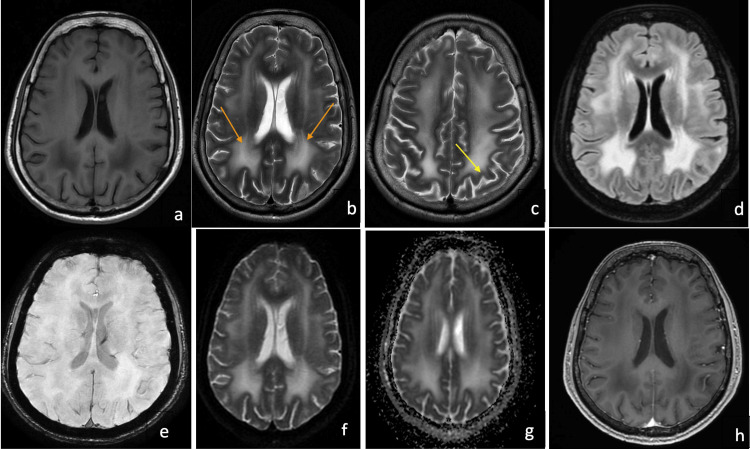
Magnetic resonance imaging (MRI) of the brain in the index case 1a. T1 weighted axial MRI of the brain showing bilateral symmetrical hypointensity in the periventricular white matter, 1b. T2 weighted axial MRI of the brain showing bilateral symmetrical hyperintensities in the periventricular white matter (orange arrows), 1c. T2 axial MRI brain highlighting the involvement of the subcortical U-fibers (yellow arrow), 1d. FLAIR hyperintensities noted in the bilateral periventricular white matter, 1e. Susceptibility-weighted imaging (SWI) showed no evidence of microbleeds, 1f. and 1g. Diffusion-weighted imaging (DWI) and attenuation diffusion coefficient (ADC) images demonstrate hyperintense signals in the periventricular white matter suggesting a T2 shine-through effect (probably due to vasogenic edema resulting from hyperpermeability of blood capillaries secondary to LAMA2 protein defect), 1h. T1 contrast images demonstrate the lack of contrast enhancement in the white matter lesions.
